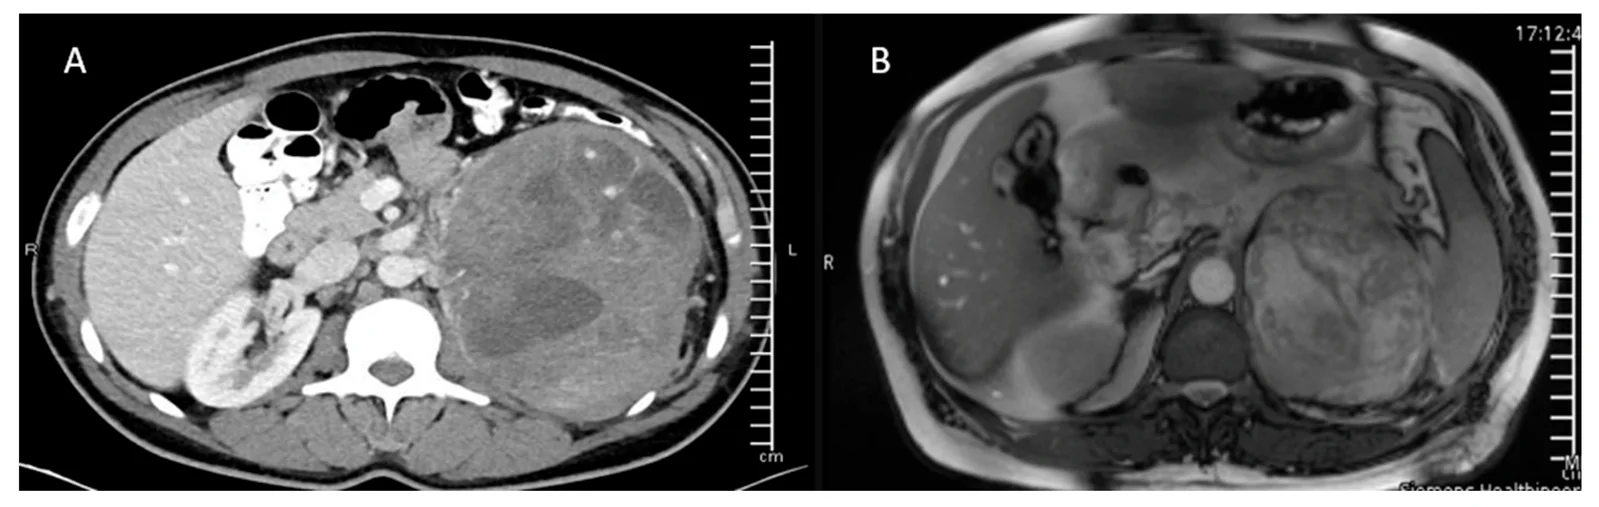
(A): CT abdomen: large mass most likely representing a left adrenal carcinoma 16 × 14 cm; however, retroperitoneal sarcoma remains a possibility. (B): MRI adrenal with contrast: A 15 cm lesion of the left adrenal gland showing hemorrhagic signal intensity and numerous vessels traversing the lesion (Case
